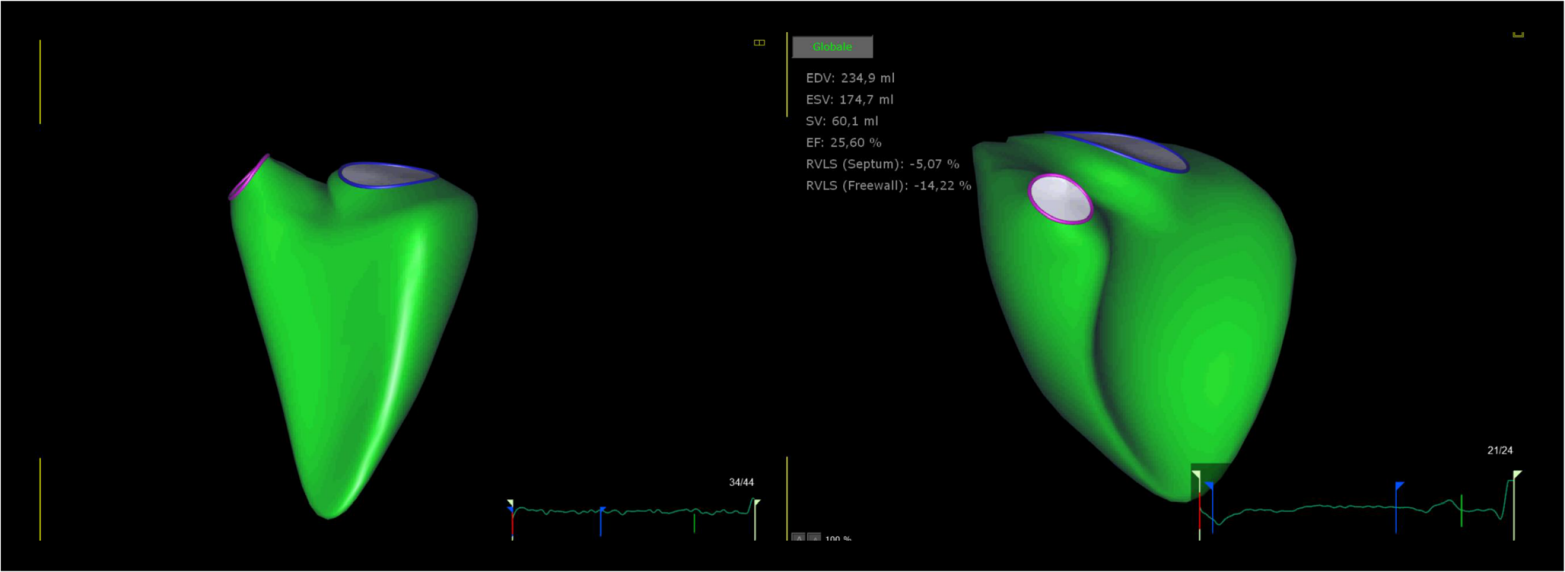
Morphological and functional difference between a healthy right ventricle and an impaired RV by 3D-echocardiography. The image on the left shows a healthy right ventricle (RV) that has a preserved triangular morphology, whereas the image on the right side shows a failing RV, with a markedly altered morphology and an impaired function, as indicated by the echocardiographic parameters listed in the picture. EDV, end-diastolic volume; EF, ejection fraction; ESV, end-systolic volume; RVLS, right ventricular longitudinal strain; SV, stroke volume
